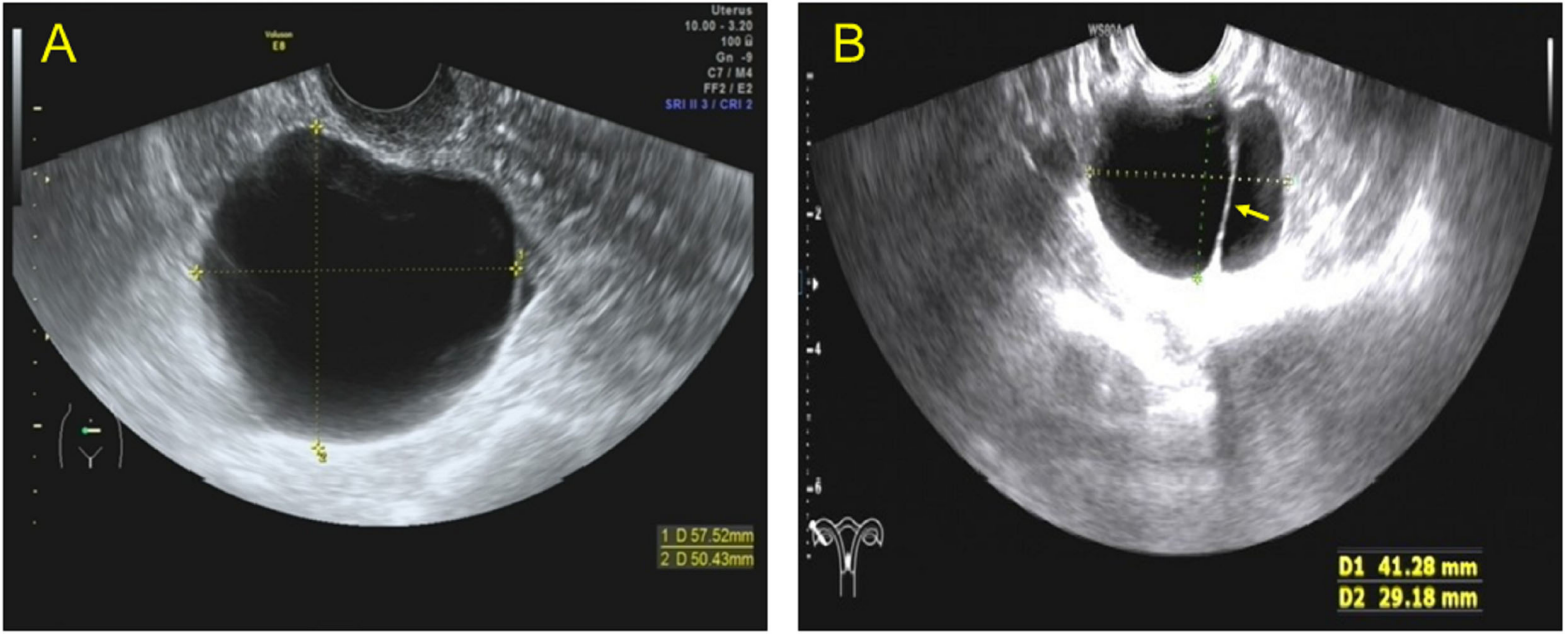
Transvaginal ultrasound images showing the cystic type lesions of benign Brenner tumor. (A) Transvaginal ultrasound (TVU) showing a case of unilocular lesions with thin walls. (B) TVU showing a case of cystic lesions with thin separation in the right ovary.
